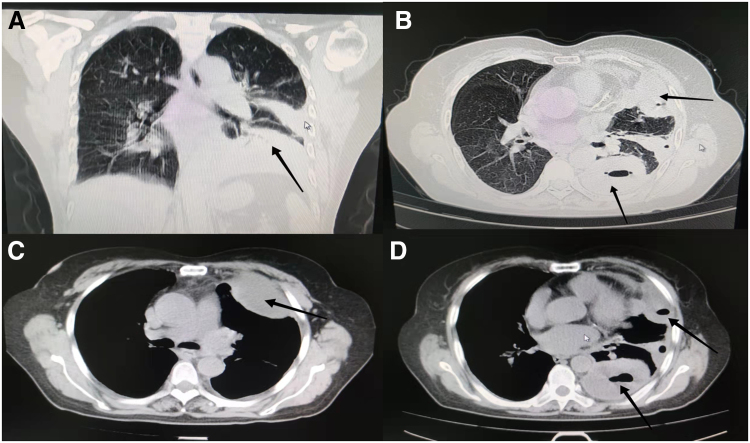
Imaging findings of loculated pleural effusion. (A) Chest radiograph shows a left-sided loculated pleural effusion (black arrows). (B) Chest CT lung window showing empyema (black arrows). (C and D) Mediastinal window shows a left-sided loculated pleural effusion (black arrows). CT = computed tomography.
